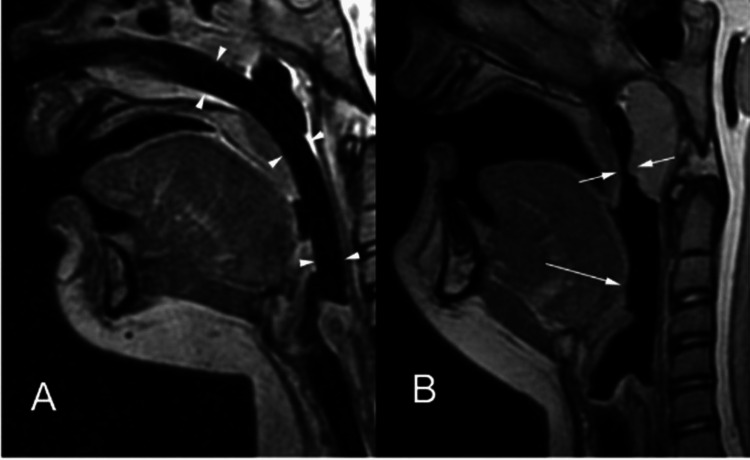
Airway depiction in MRI. MRI accounts for better soft tissue evaluation, especially in the airway. (A) depicts the airway being divided into the nasopharynx, oropharynx, and hypopharynx, whereas (B) depicts a constricted airway.
